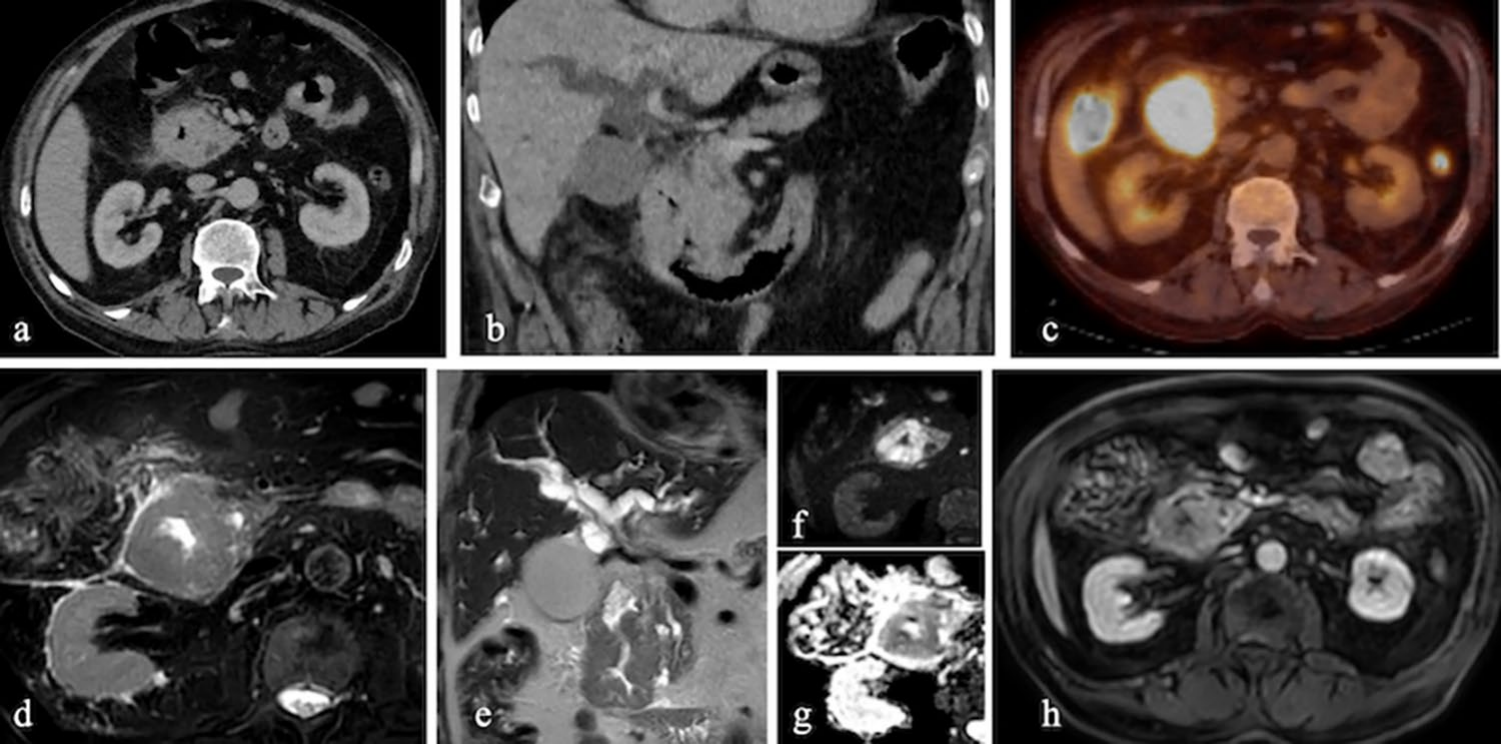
IV contrast enhanced a. Axial, b. Coronal CT, c. FDG-PET CT fusion images and d. Axial T2-weighted e. Coronal T2-weighted f. Diffusion weighted, g. ADC map and h. IV contrast enhanced fat saturated T1-weighted MR images of a 53-year-old female patient with duodenal mass diagnosed as adenocarcinoma. There is concentric wall thickening with heterogeneous enhancement. There is prominent FDG uptake. Lesion has low signal in T2-weighted imaging and shows diffusion restriction. As a result of choledochal involvement, there is dilatation in intrahepatic biller system
